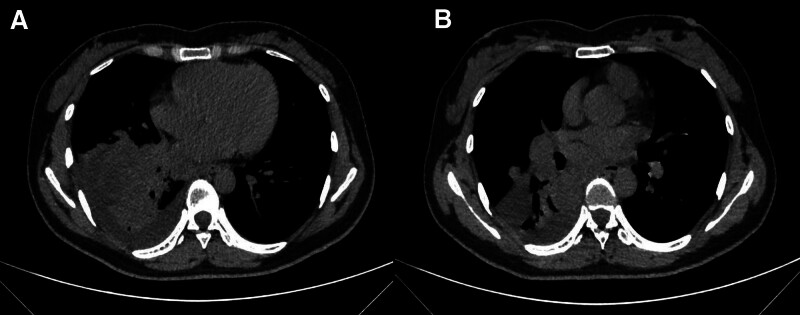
January 5, 2022. Lungs computed tomography: malignant tumor of the right lung fuller than before. Right hilar and mediastinal lymph node metastasis, fuller than before; right pleural effusion. (A) Maximum cross-section of tumor. (B) Cross-section similar to Figure 1B.
